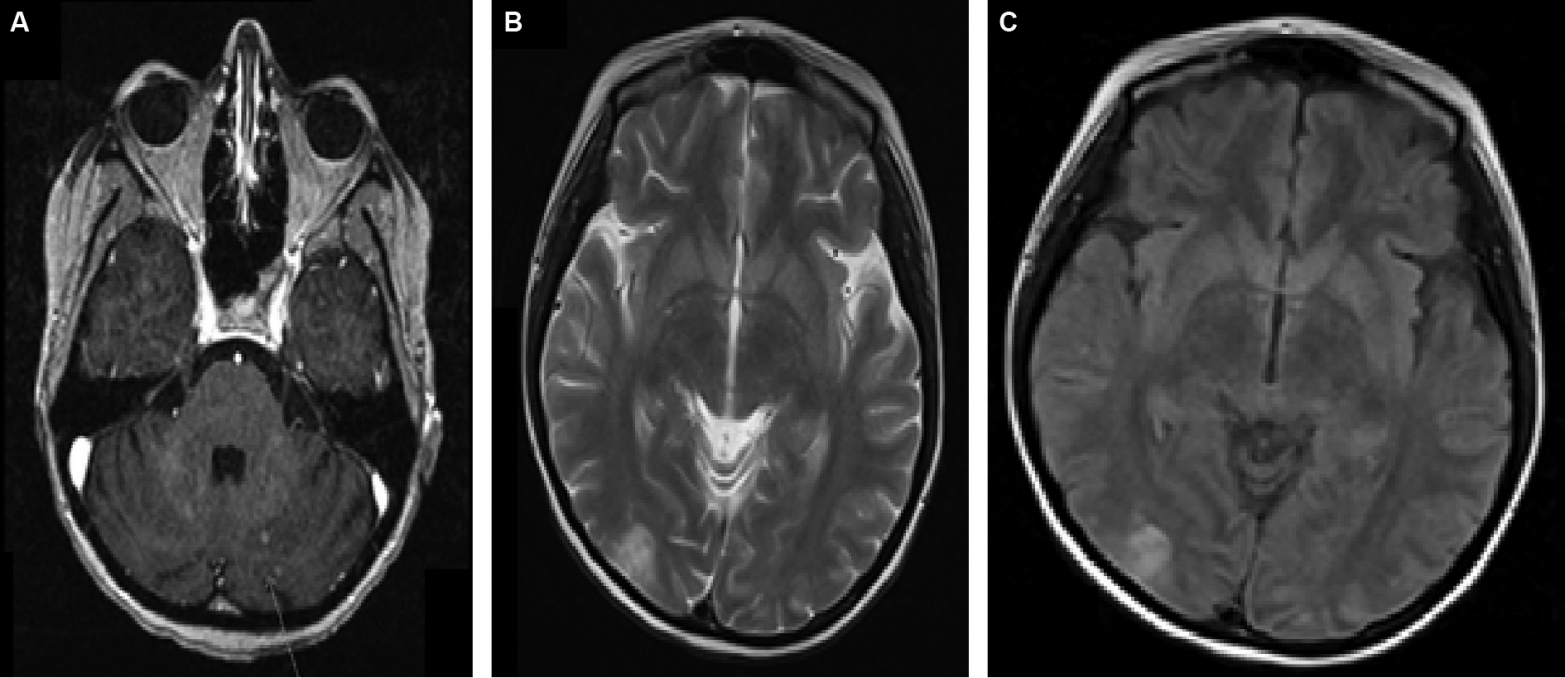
Brain MRI with contrast. (a) *T*_1_ sequence, (b) *T*_2_ sequence, (c) *T*_2_-flair sequence. These sequences show multiple bilateral *T*_2_ and flair hyperintense cortical and subcortical lesions in the parietal and occipital lobes, as well as in the cerebellar hemispheres. Some lesions showed minimal enhancement. No significant surrounding oedema, intracranial haemorrhage, space-occupying lesions, hydrocephalus or midline shift are seen.
